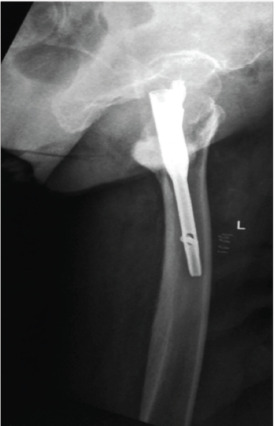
Radiograph showing anterior cortical abutment of nail tip due to excessively posterior entry point.
